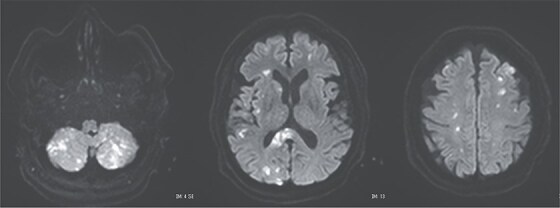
Postoperative magnetic resonance imaging showing diffuse cerebral infarctions.
